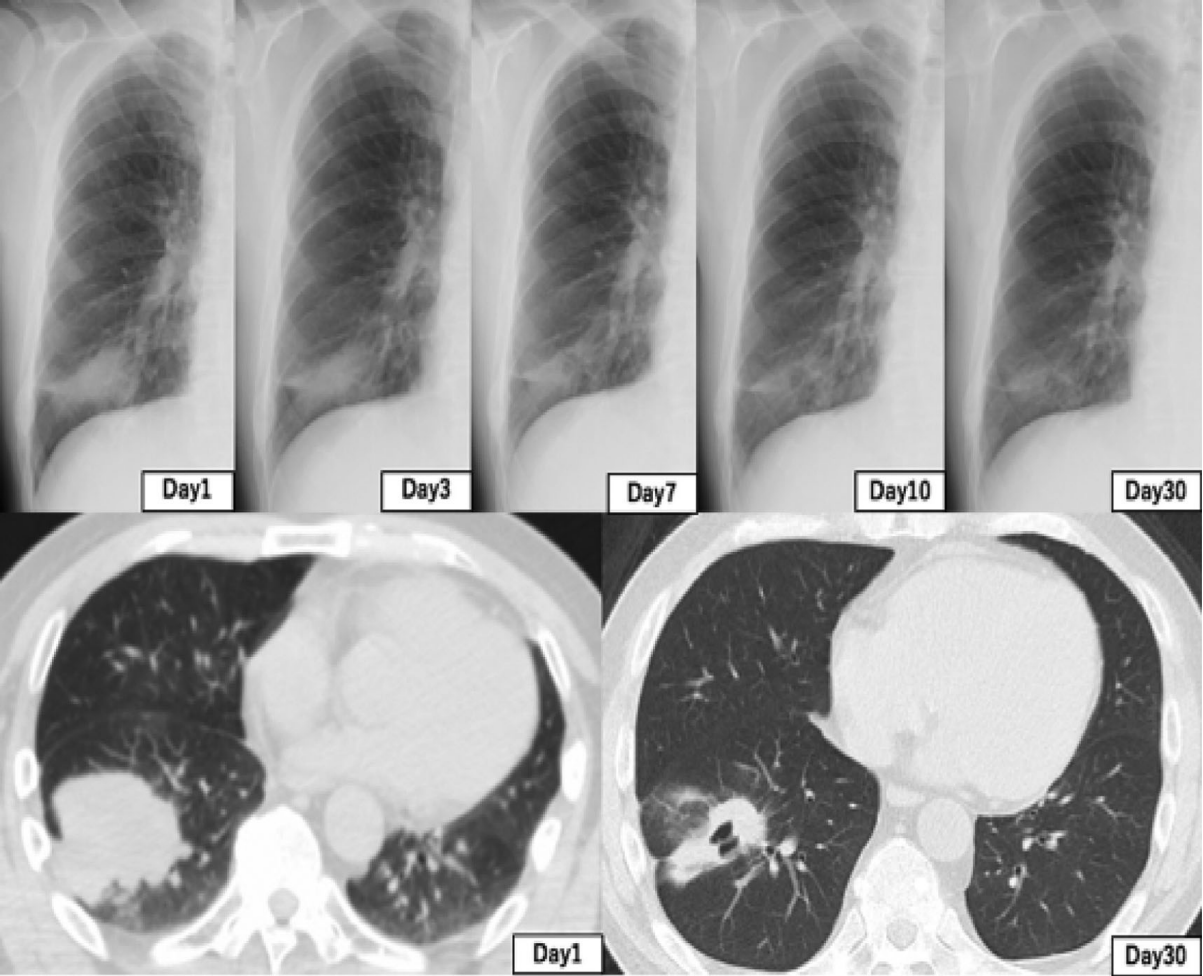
Computed tomography (CT) and chest X‐ray images showing response dynamics of the regrown primary tumor during osimertinib treatment. A decrease in tumor burden after one month was observed compared with that on day 1. The response was noticed within a few days of starting the treatment by chest X‐ray imaging.
